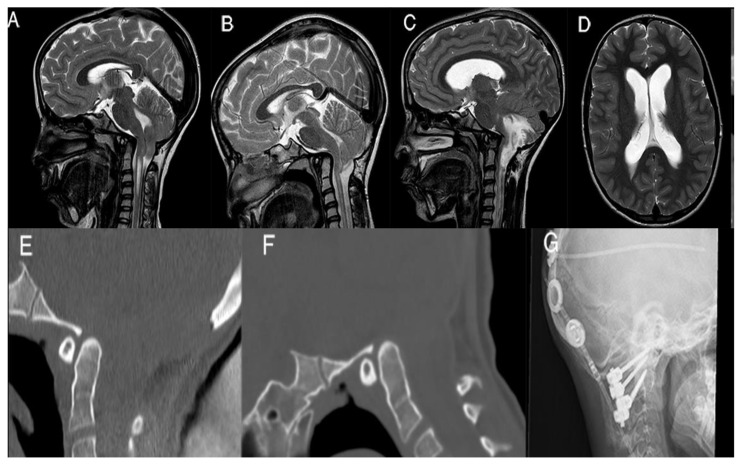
An 11-year-old girl with severe tonsil descent, hindbrain herniation, and cervical syringomyelia ((A), preoperative T2-w.i. sagittal MRI). The first MRI ((B), T2-w.i. sagittal scan) depicted flow restoration in the posterior fossa and syrinx reduction. However, six months after surgery, the child experienced the onset of acute headache and neck pain. A new MRI scan showed tonsil infarct, cerebellar swelling, and hydrocephalus ((C), T2-w.i. sagittal scan). The dynamic cervical CT scan (D,E) demonstrated slight instability. The patient underwent ventriculoperitoneal shunt positioning with a programmable valve and C1–C2 fixation ((F), as shows the postoperative X-ray lateral plan (G)).
